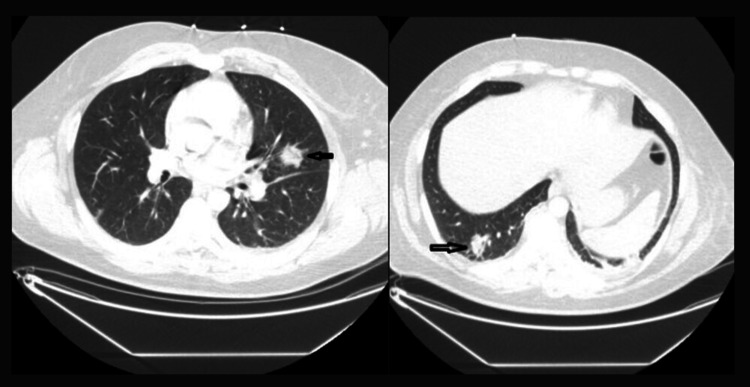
CT thorax showing septic emboli bilaterally (arrows)
